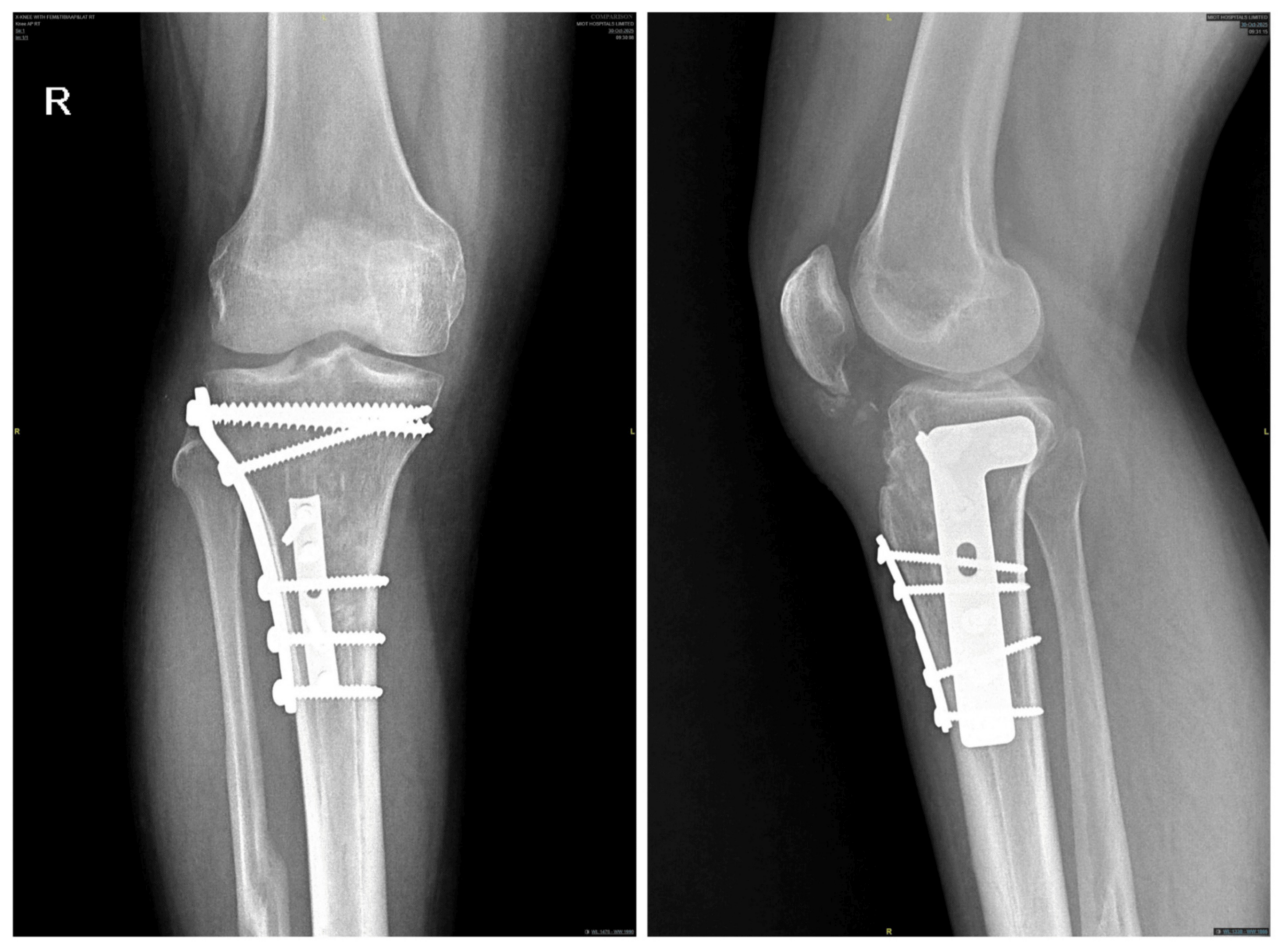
Three and half months' follow-up X-ray of the right knee showing healed fracture after internal fixation
